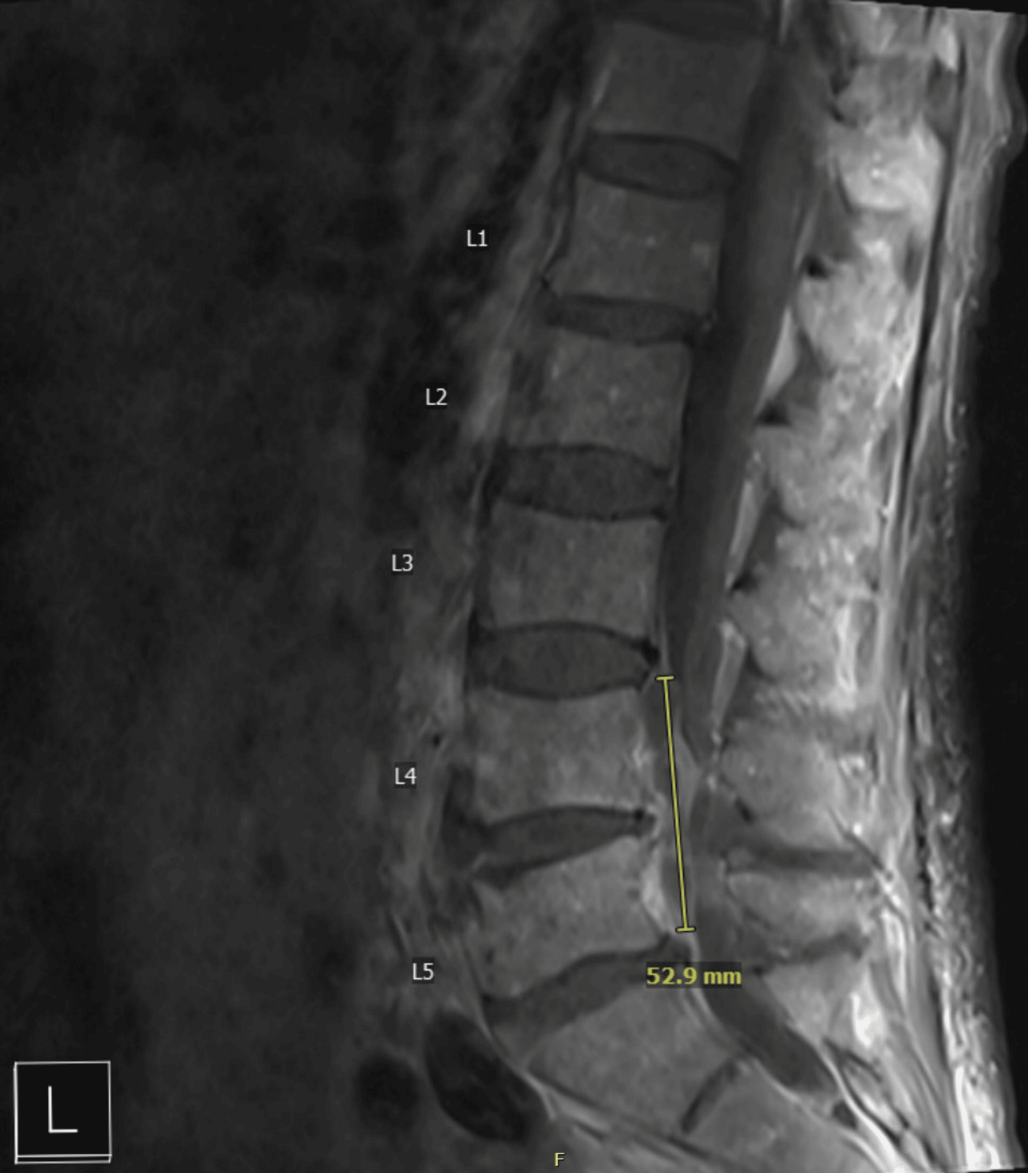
Sagittal magnetic resonance imaging (MRI) of the lumbar spine demonstrating a localized paraspinal abscess/phlegmon at the L4–L5 level. The yellow line delineates the craniocaudal extent of the abscess, measuring approximately 52.9 mm.
